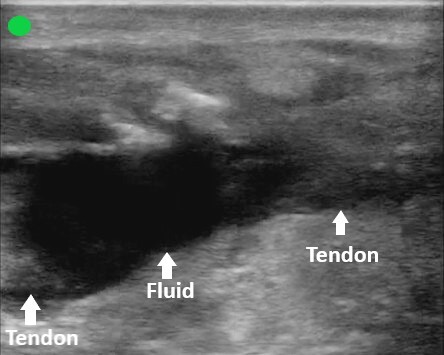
Sagittal view of the proximal Achilles tendon. Point-of-care ultrasound image obtained with a high-frequency linear probe with the probe marker directed cephalad. A large anechoic fluid collection is present between two distinct ends of the ruptured tendon.
